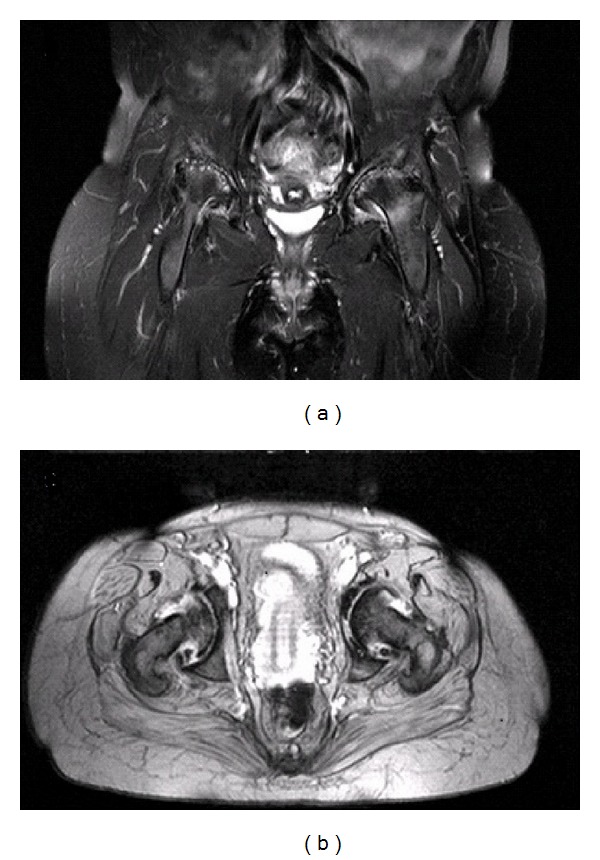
(a) MRI coronal FS view of the pelvis and (b) MRI axial ME 2D view of the pelvis.
